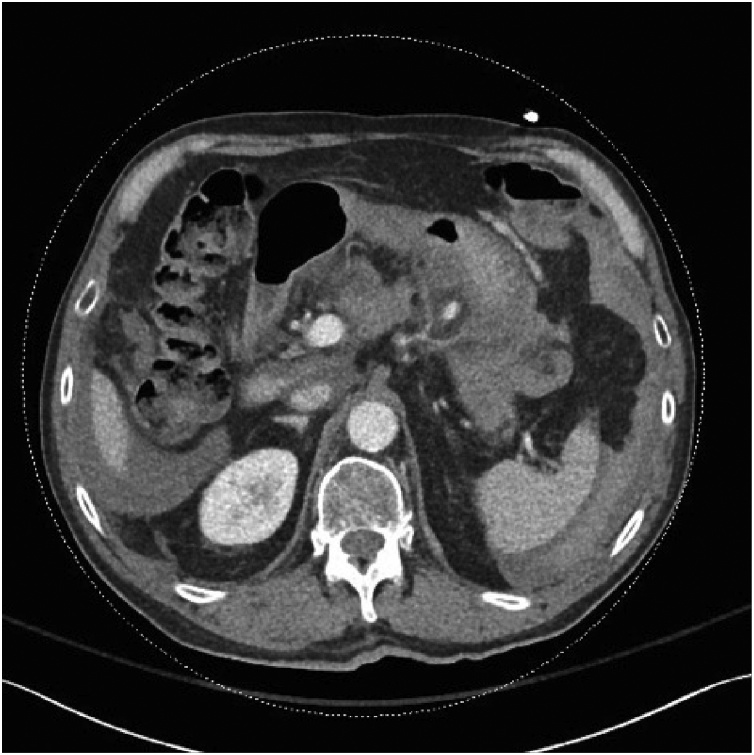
CT Angiogram in the portal venous phase demonstrating stable pseudoaneurysms of the left gastric artery and interval development of left gastric artery attenuation in keeping with a focal dissection and intramural thrombus.
